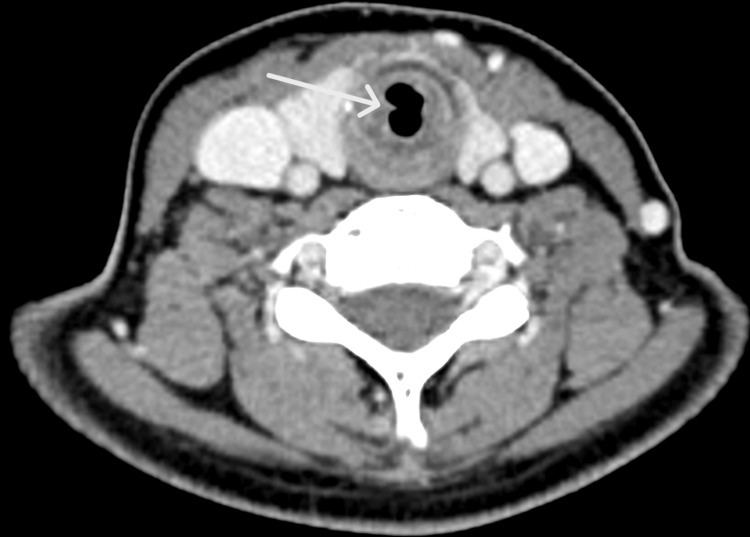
Additional axial CT slice demonstrating the extent of the stenosis
